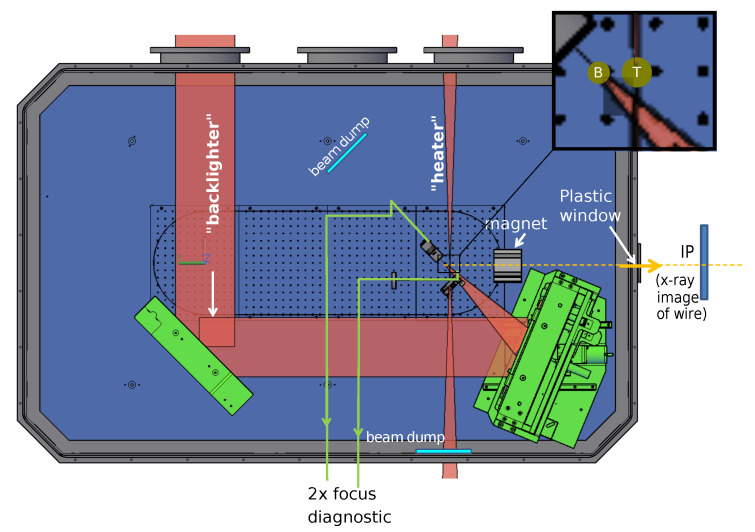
Measurement setup at Gesellschaft für Schwerionenforschung (GSI). The laser beam is split into backlighter and heater. The backlighter is shot at a tungsten wire for generating X-rays. The heater can optionally be shot at an object for generating shock waves or explosions. The inset shows a zoom-in on the tungsten backlighter wire (B) and the titanium wire (T) positions, depicted as green circles. In the presented measurement the heater is not shot at the titanium wire and the cold titanium wire is imaged. The images are obtained with imaging plates. The magnet is used to divert electrons, which occur due to the explosion of the wire and cause noise in the acquired images.
